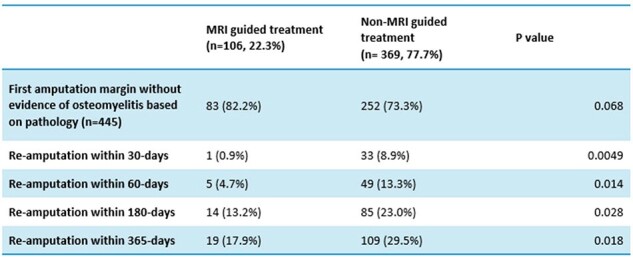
Bivariate analysis of re-amputations MRI=magnetic resonance imaging
